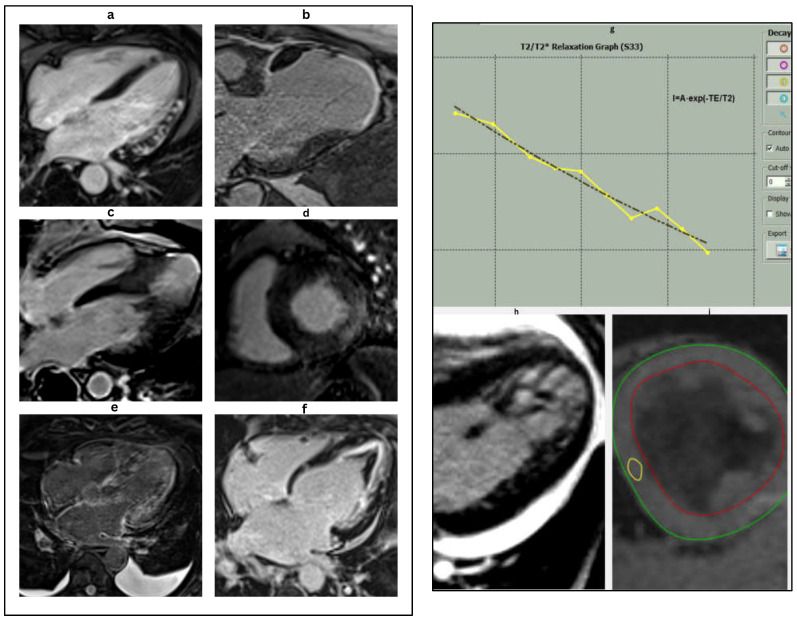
Characteristic cardiac MRI findings in different HF etiologies. (a): Four chamber delayed enhancement CMR in a patient with myocarditis. Late gadolinium enhancement (LGE) is seen in the mid-myocardial to subepicardial layers of the anterolateral wall, a typical non-ischemic pattern of injury. These findings are consistent with inflammatory myocardial involvement, commonly seen in viral or immune-mediated myocarditis. (b): Cardiac magnetic resonance (CMR) image demonstrating ischemic cardiomyopathy. The image reveals marked apical thinning with transmural late gadolinium enhancement (LGE) in the left anterior descending (LAD) artery territory, consistent with chronic infarction and scar formation. These findings are typical of prior anterior myocardial infarction with associated adverse remodeling. (c): 4-ch delayed enhancement CMR in apical HCM showing mid-septal LV hypertrophy and apical scar. (d): Short-axis LGE image revealing inferolateral mid-myocardial to epicardial lateral wall hyper-enhancement and concentric LV hypertrophy in Fabry’s disease. (e): Cardiac magnetic resonance imaging (CMR) in the 4-chamber view with late gadolinium enhancement (LGE) in a patient with cardiac amyloidosis. The image demonstrates diffuse, global subendocardial, and transmural late gadolinium enhancement, a hallmark feature of cardiac amyloid infiltration. Note the difficulty in nulling the myocardial signal, another characteristic finding in cardiac amyloidosis. (f): Cardiac magnetic resonance (CMR) image in a patient with endomyocardial fibrosis (EMF). The four-chamber late gadolinium enhancement (LGE) image demonstrates apical scarring with superimposed thrombus in the left ventricle, characteristic of EMF, and reflects chronic fibrotic remodeling leading to restrictive physiology. (g): T2* relaxation curve showing exponential signal decay over time, characteristic of myocardial iron overload. The yellow line represents the T2 relaxation process, which describes the decay of transverse magnetization due to interactions between nearby spins. The black line shows the T1 relaxation process, which depicts the recovery of longitudinal magnetization as spins realign with the external magnetic field. These curves are fundamental for differentiating tissue types in MRI based on their distinct relaxation times. The rapid drop in signal intensity reflects shortened T2* values due to increased magnetic susceptibility from iron deposition. Quantitative T2* analysis is essential for assessing the severity of cardiac siderosis, particularly in conditions such as thalassemia major or hemochromatosis. (h): LV cine image displaying prominent trabeculations and deep intertrabecular recesses, predominantly in the apical and lateral segments characteristic of left ventricular non-compaction (LVNC). (i): Short-axis cardiac magnetic resonance (CMR) image of the mid-left ventricle used for T2* mapping. The green line represents the outer boundary of the myocardial wall, while the red line indicates the inner endocardial lining. The region of interest (ROI) (in yellow) is typically placed in the interventricular septum to quantify myocardial T2* relaxation times. This technique is essential for detecting and monitoring myocardial iron overload, with lower T2* values indicating increased iron deposition.
